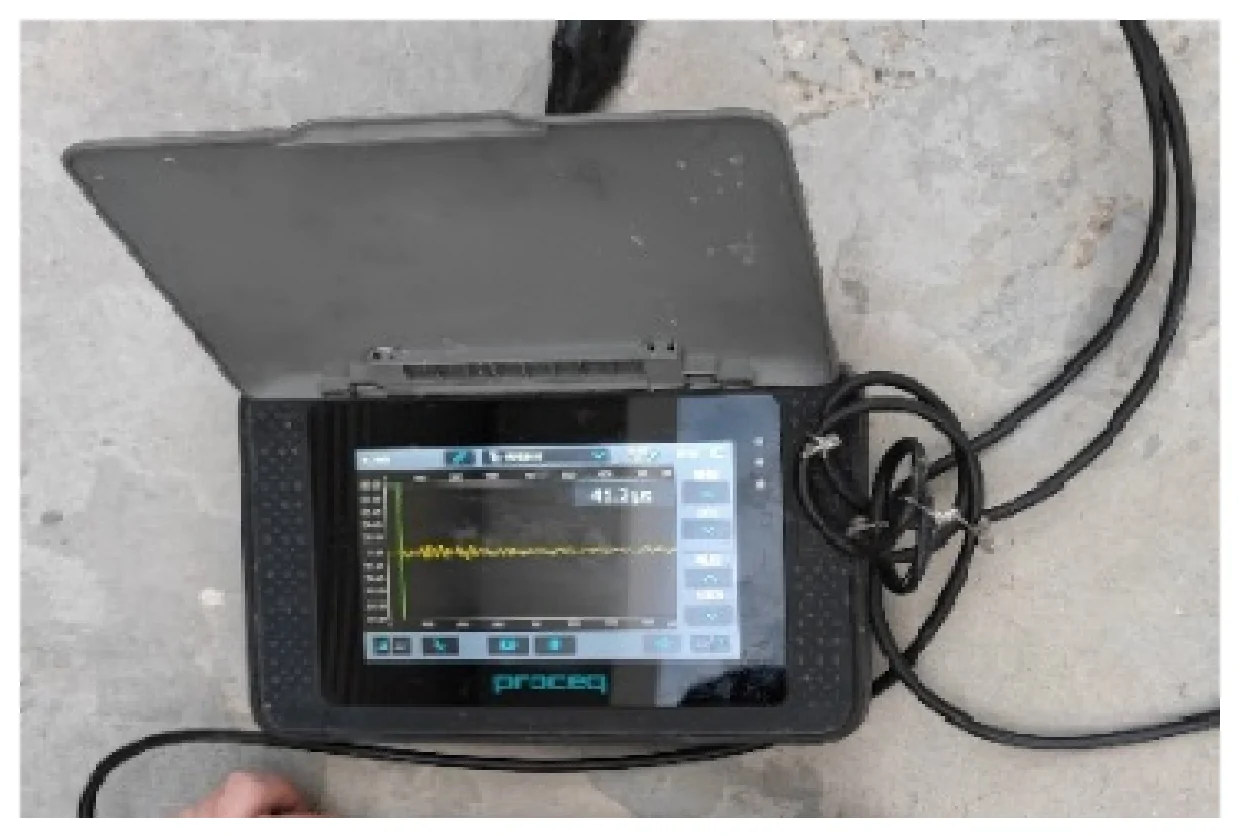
Ultrasonic nondestructive tester.
